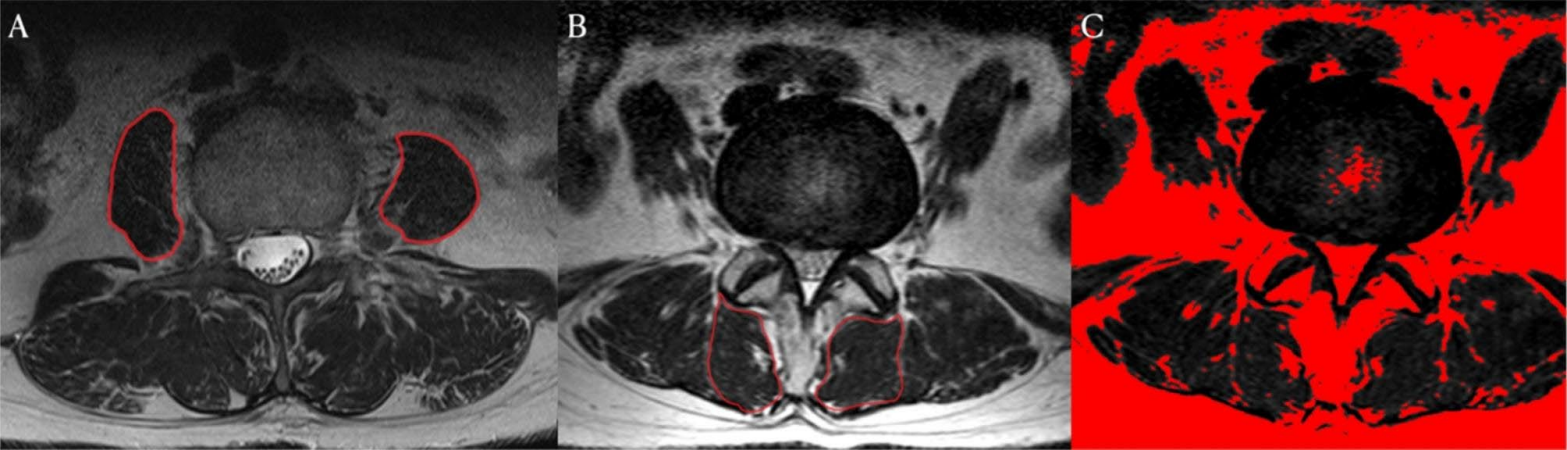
Psoas and multifidus measurement using spine MRI. The psoas and multifidus muscles were drawn directly on axial T2-weighted magnetic resonance (MR) images. (A) The psoas muscle’s cross-sectional area (CSA) was manually selected at the upper level of the L3 vertebral body. (B) CSA of the multifidus muscle is marked at L4–5 and L5–S1 levels, respectively. (C) The fat area of muscles on the T2-weighted MR image was converted to red using the pseudo-coloring technique in the software based on the threshold. The percentage of muscle fibers (PMF) in the multifidus muscle was calculated by subtracting the red area from the CSA and multiplying it by 100
